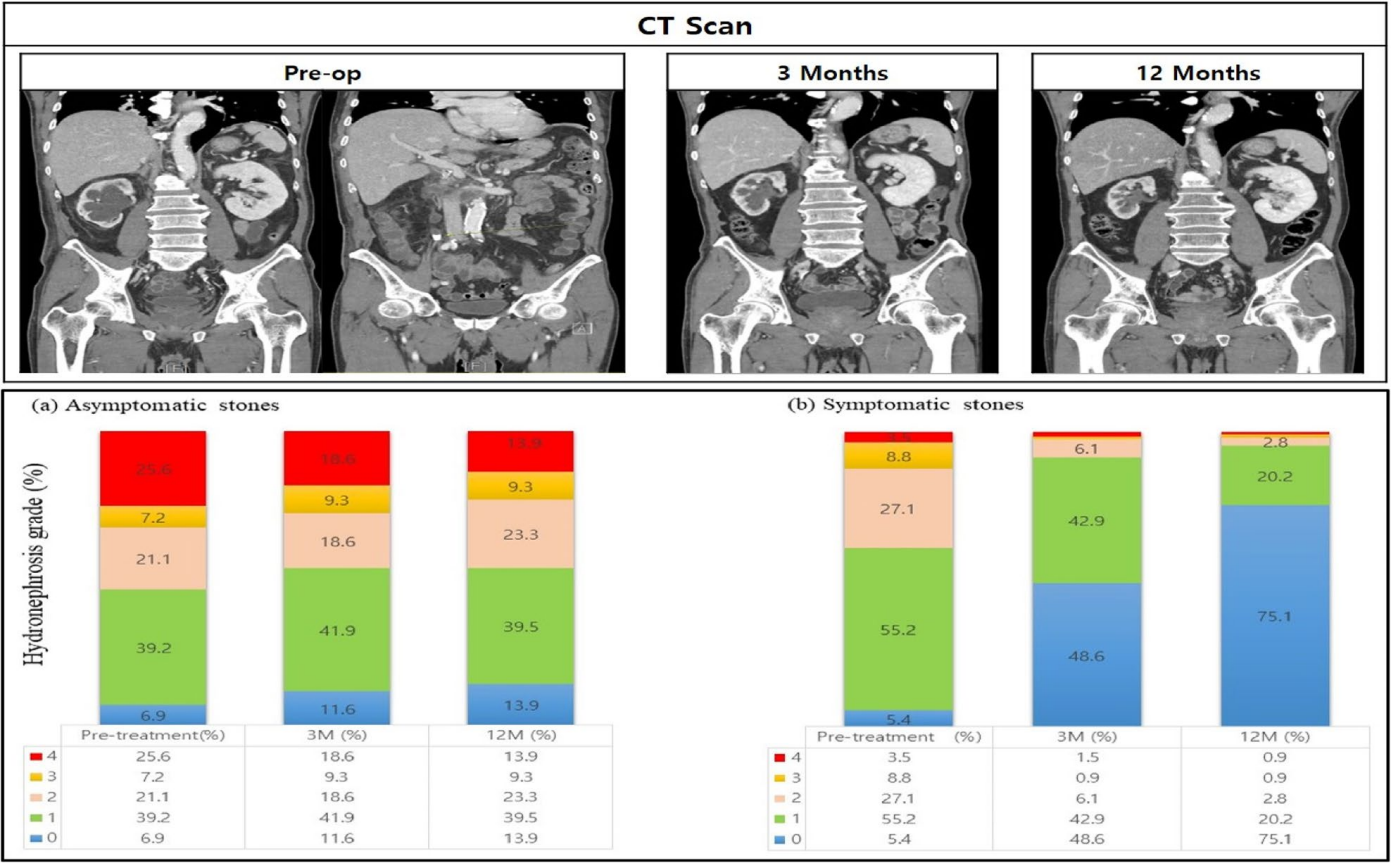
Changes in hydronephrosis grade estimated by serial computed tomography after stone treatment.
